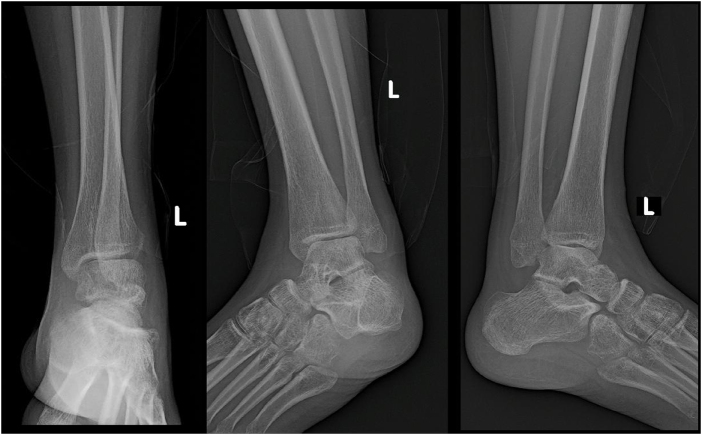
Preoperative X-ray: This image shows a posterior dislocation of the distal fibula behind the tibia, with no visible fracture lines..
